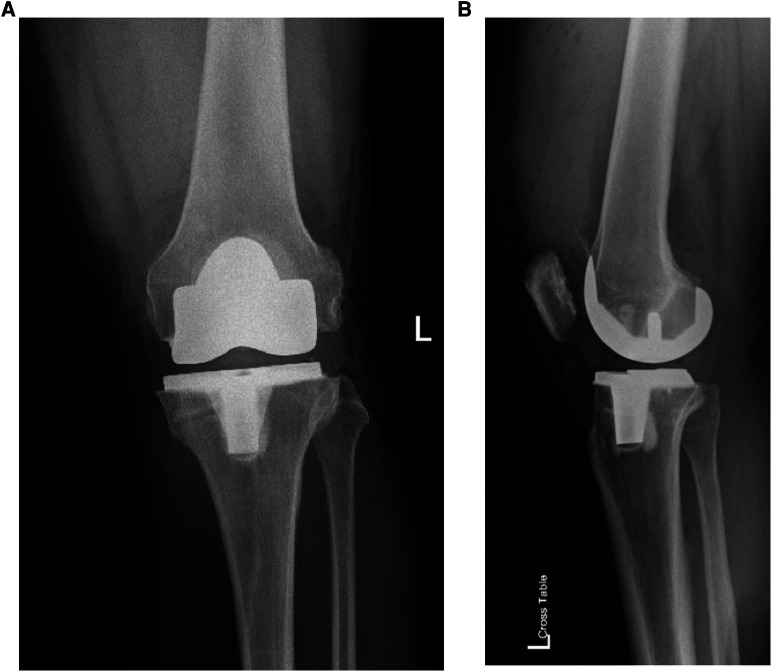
(A) anteroposterior and (B) lateral radiograph of the same patient demonstrates a balanced left TKA.
